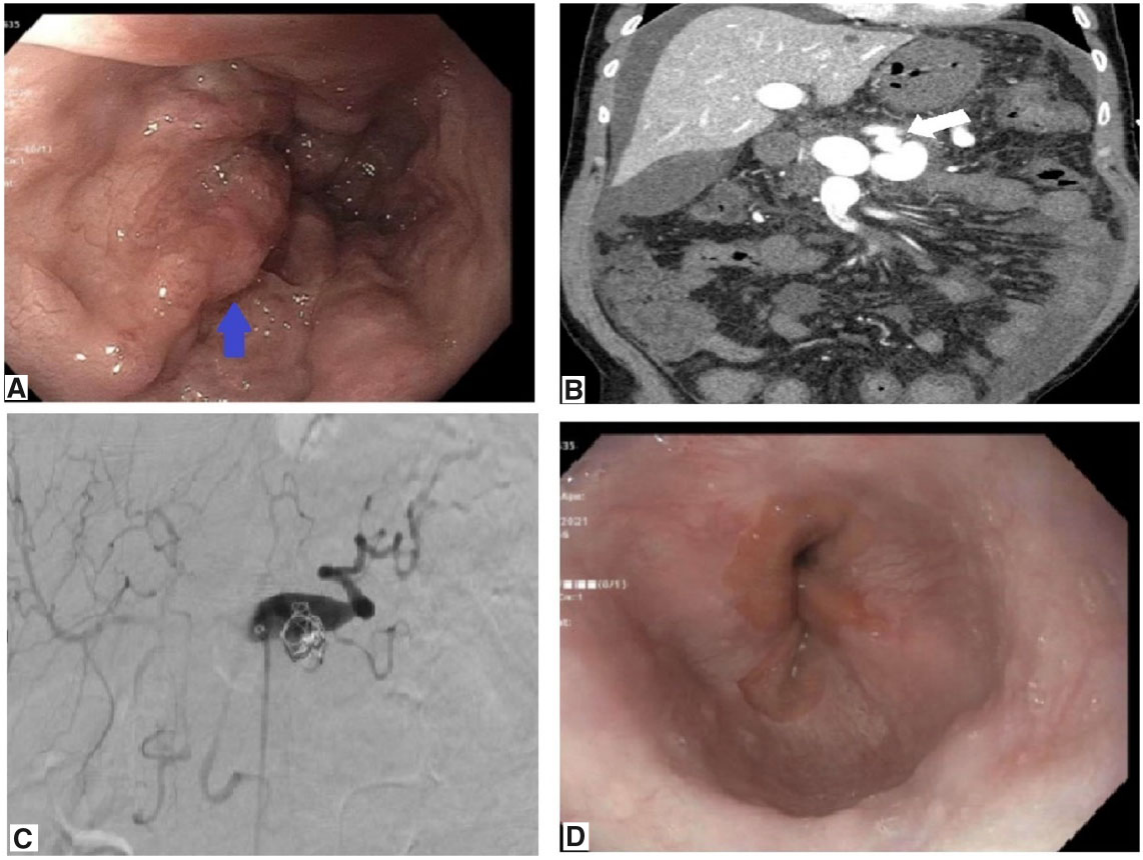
Endoscopy and computed tomography angiography on admission and 2 months later. (A) Endoscopy on initial presentation showing large esophageal varices (blue arrow). (B) Computed tomography angiography on initial presentation showing splenic arteriovenous fistula (white arrow). (C) Percutaneous transcatheter embolization of the arteriovenous fistula and the pseudoaneurysm using 12-mm Amplatzer plug and coil. (D) Repeated endoscopy showing resolution of the esophageal varices after 2 months.
